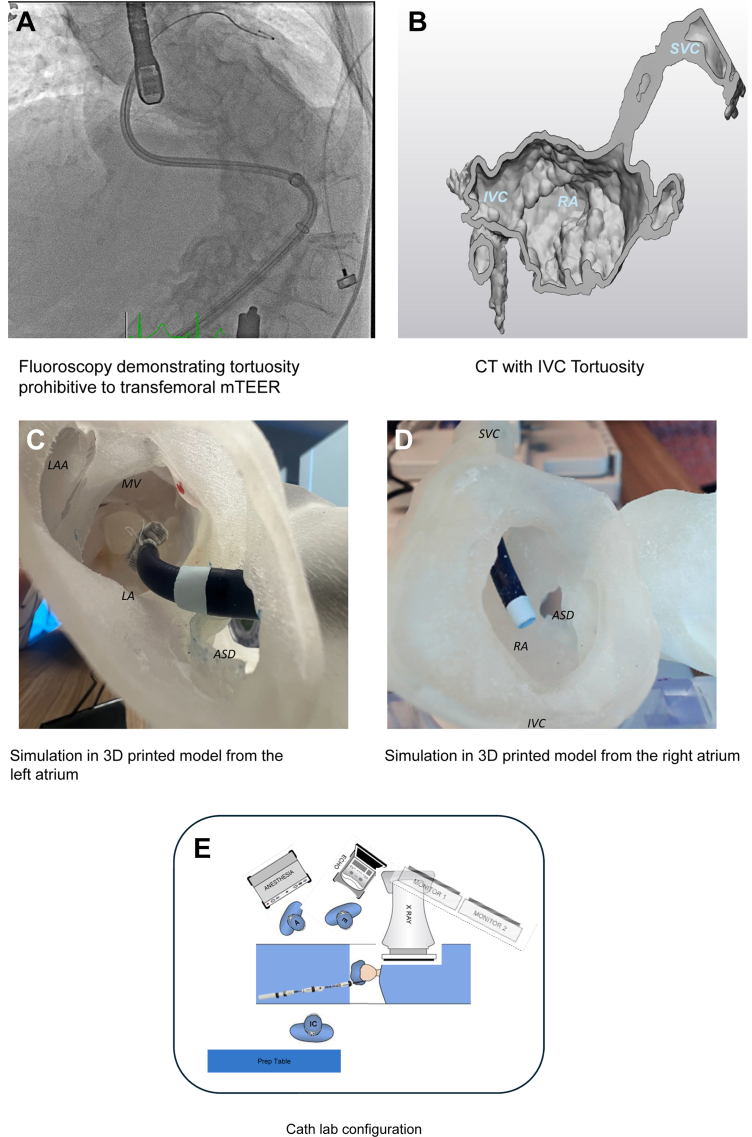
Preprocedural Planning (A) Fluoroscopy demonstrating severe scoliosis and tortuosity of the inferior vena cava from a right common femoral vein approach. (B) Computed tomography–generated 3-dimensional image demonstrating the IVC tortuosity as well as the SVC trajectory. (C) A 3-dimensional printed model demonstrating right transjugular access with the delivery sheath and device from the left atrium. (D) A 3-dimensional printed model demonstrating right transjugular access with the delivery sheath and device from the right atrium. (E) Catheter laboratory configuration to perform internal jugular vein mitral transcatheter edge-to-edge repair. A = anesthesiologist; ASD = atrial septal defect; E = echocardiologist; ECHO = echocardiography; IC = interventional cardiologist; IVC = inferior vena cava; LA = left atrium; LAA = left atrial appendage; MV = mitral valve; RA = right atrium; SVC = superior vena cava.
